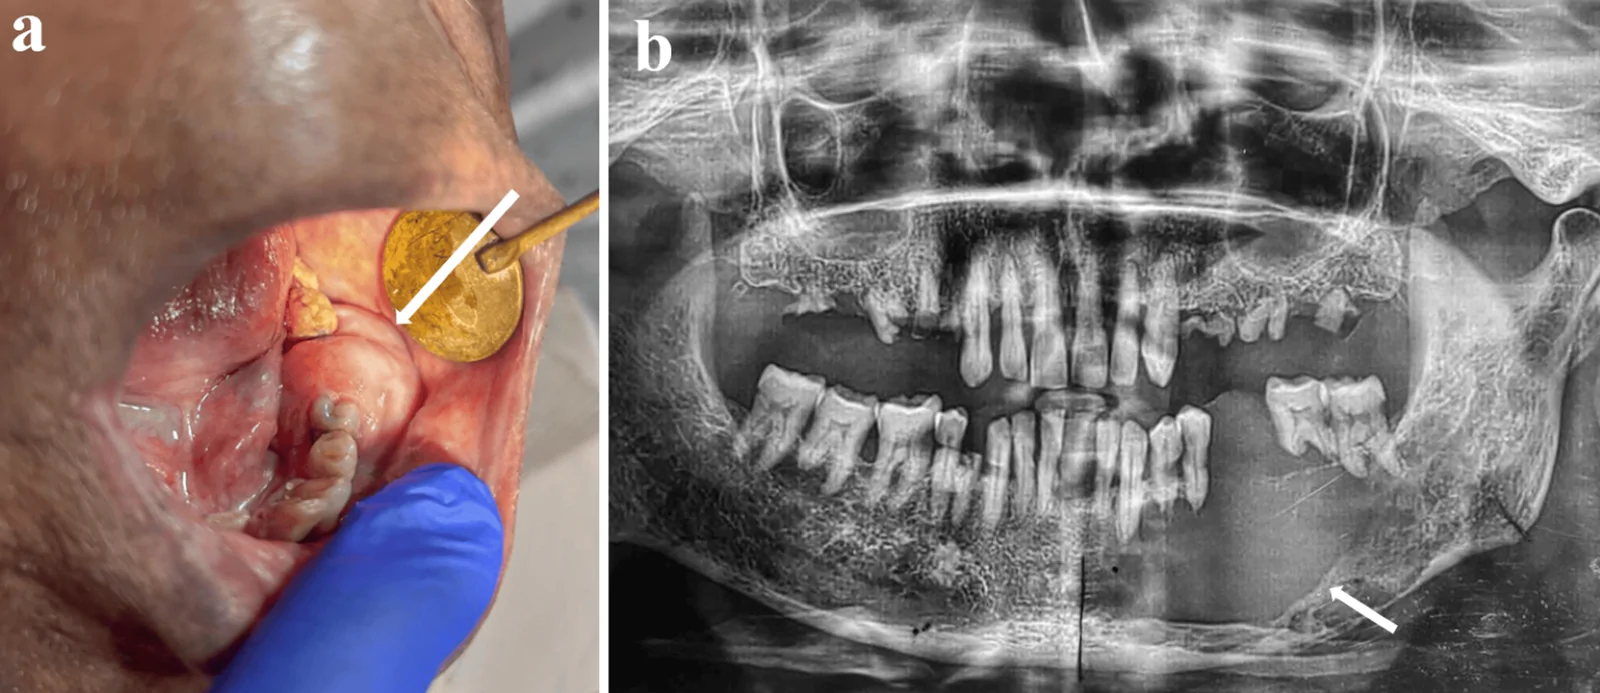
Initial clinical and radiographic presentation of mandibular plasmacytoma. (a) Intraoral photograph demonstrating a smooth, sessile swelling involving the left posterior mandibular alveolar ridge (arrow). (b) Orthopantomogram showing a well-defined unilocular osteolytic lesion extending from the left mandibular body to the angle with cortical destruction and expansion (arrow), corresponding to the site of the plasma cell neoplasm.
